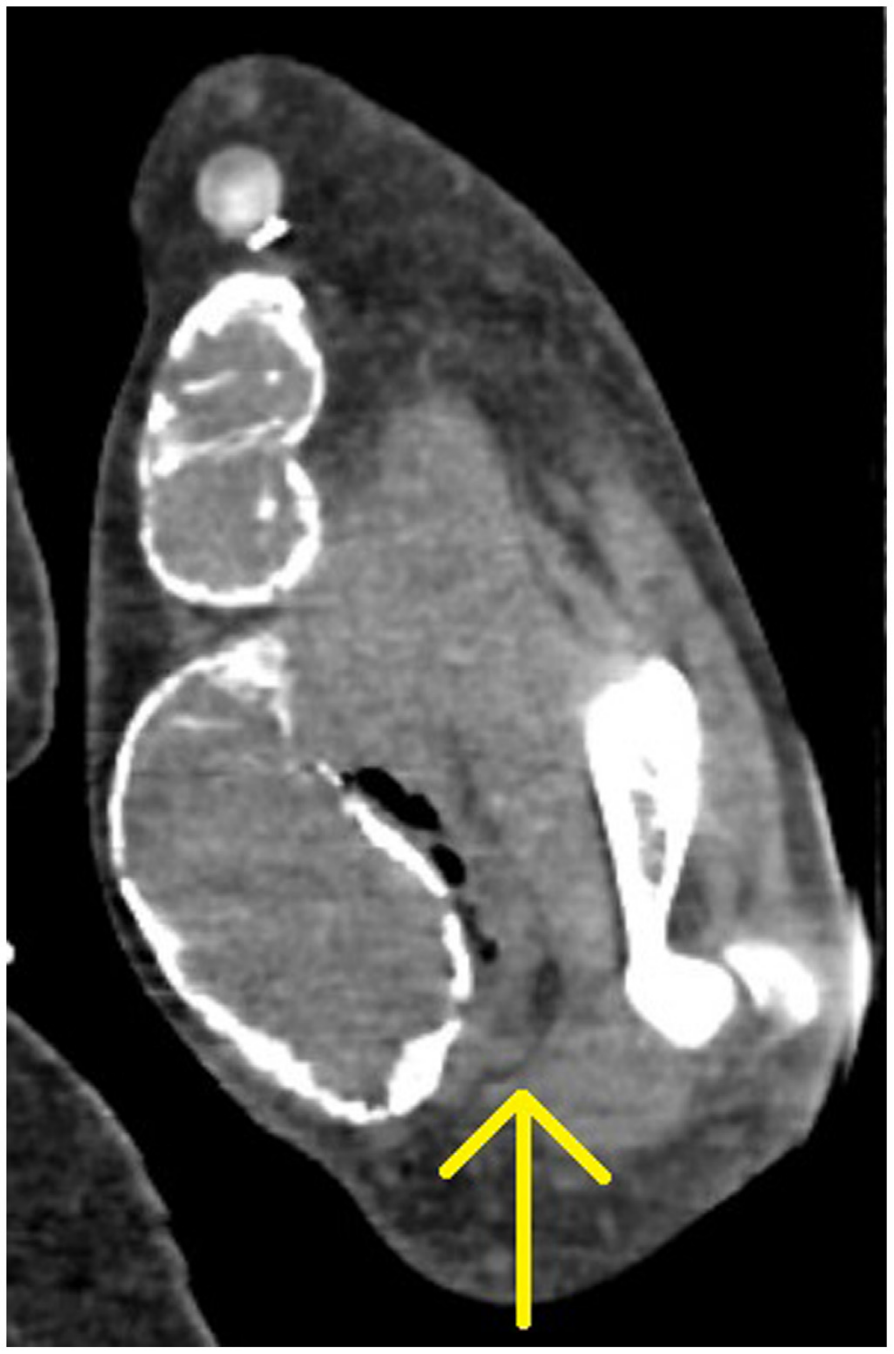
Coronal cut from computed tomography done on admission showing gas and fluid surrounding the patient’s left upper extremity arteriovenous fistula (AVF). Yellow arrow showing fluid/air collection.
